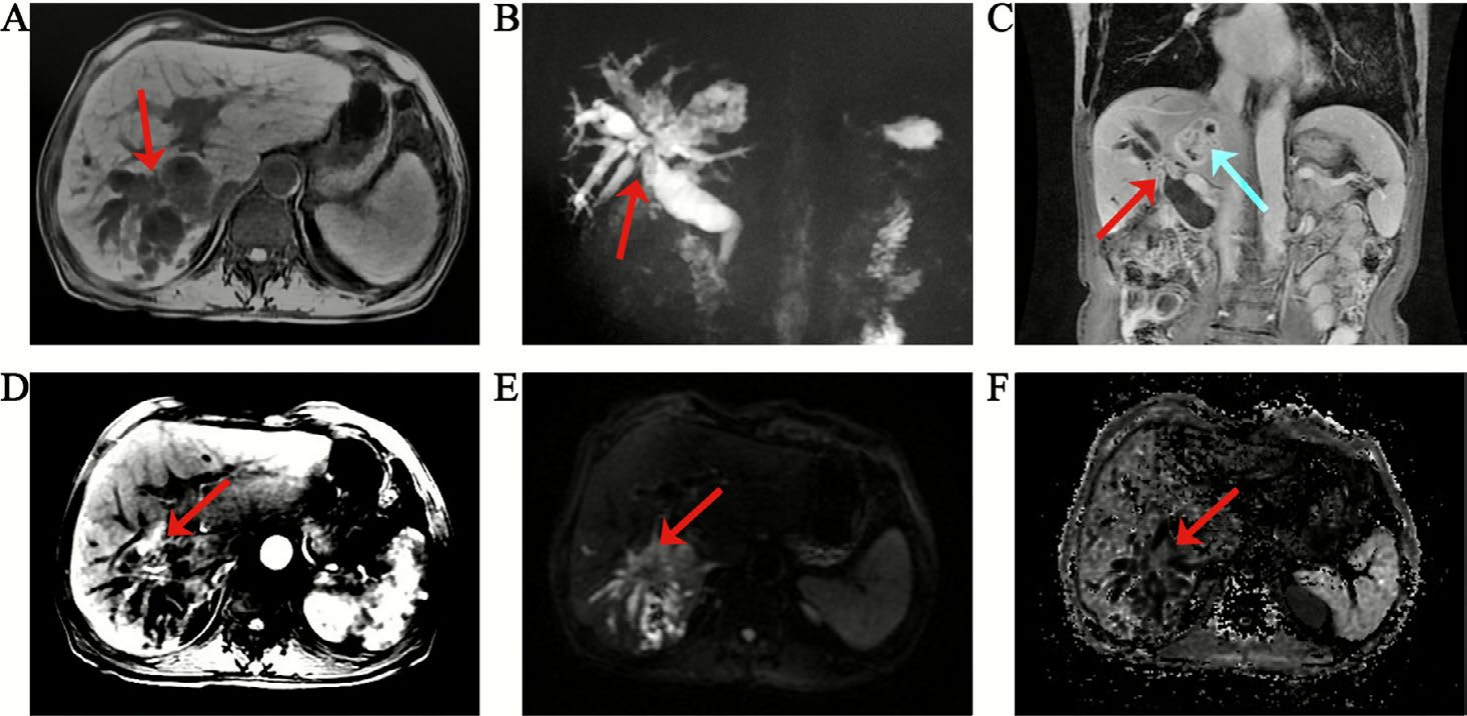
Magnetic resonance imaging (MRI), magnetic resonance cholangiopancreatography (MRCP), and contrast enhanced MRI. (A) MRI T2‐weighted image, honeycomb‐like mixed signal shadow was found in the right sector (segments V to VIII) near the hepatic hilum, and patchy high signal shadow was seen at the edge. (B) MRCP, the intrahepatic bile ducts of the right sector (segments V to VIII) were dilated to varying degrees with multiple filling defects; (C) MRI Enhanced coronal plane, red arrow: Hilar bile duct stenosis and thickening, blue arrow: Honeycomb‐like mixed signal shadow in the right sector (segments V to VIII) of the liver; (D) T2‐weighted image, patchy high signal shadow at the edge of the right sector (segments V to VIII) of the liver; (E) DWI, slightly high signal; (F) ADC, no significant decrease was observed.
